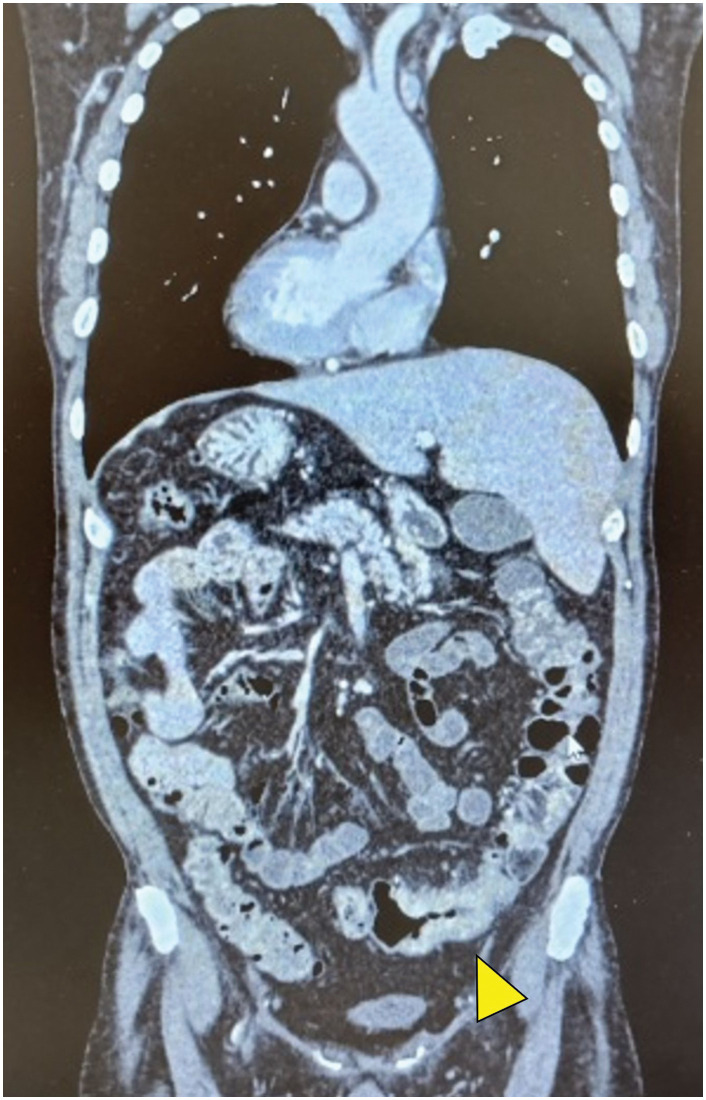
Contrast-enhanced CT showing complete mirror-image anatomy consistent with situs inversus totalis. The liver is on the left and the spleen on the right. CT image demonstrating wall thickening of the sigmoid colon (arrowhead), corresponding to the primary tumor.
